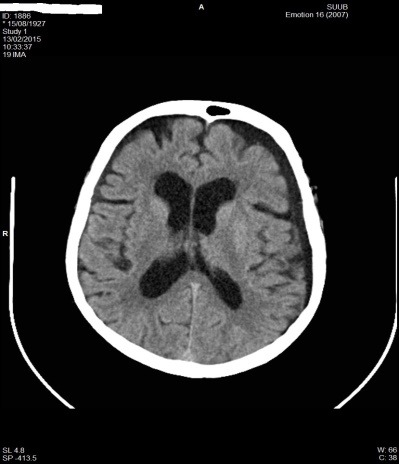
CT aspect with a left F-T hygroma in patient P.M. of 87 years old
